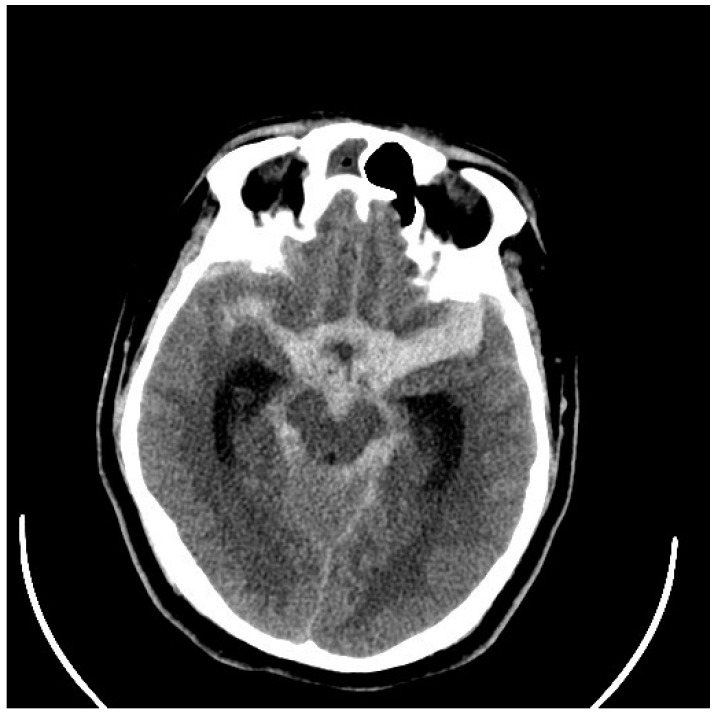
CT scan through the basal subarachnoid cisterns showing thick, hyperdense clots in a 31-year-old man who was drowsy and confused after a sudden onset headache with vomiting (Hunt and Hess and WFNS grade III). Note the thickest clot is in the left Sylvian fissure surrounding the left MCA (The following figures are all from the same patient).
